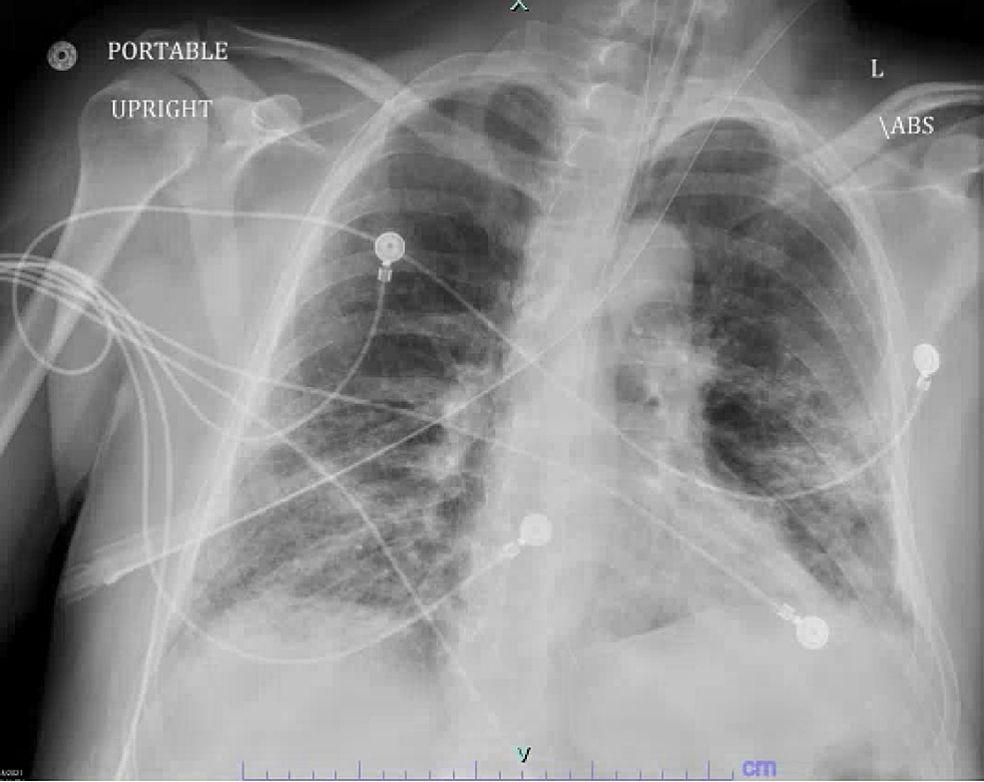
Chest X-ray on initial presentation showing bilateral diffuse infiltrates consistent with COVID-19
